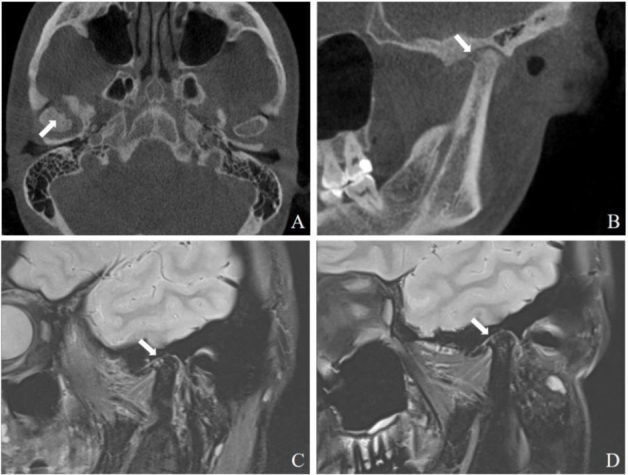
A: Axial view of CBCT shows osteophyte and surface erosion in the anterior bevel of the right condyle (arrow). B: Oblique sagittal view of CBCT shows osteophyte and surface erosion in the anterior bevel of the right condyle (arrow). C: MRI shows subcortical cyst in the right joint fossa and condyle (arrow). D: MRI shows reduction of cystic lesions in the right articular fossa and condyle after treatment (arrow).
